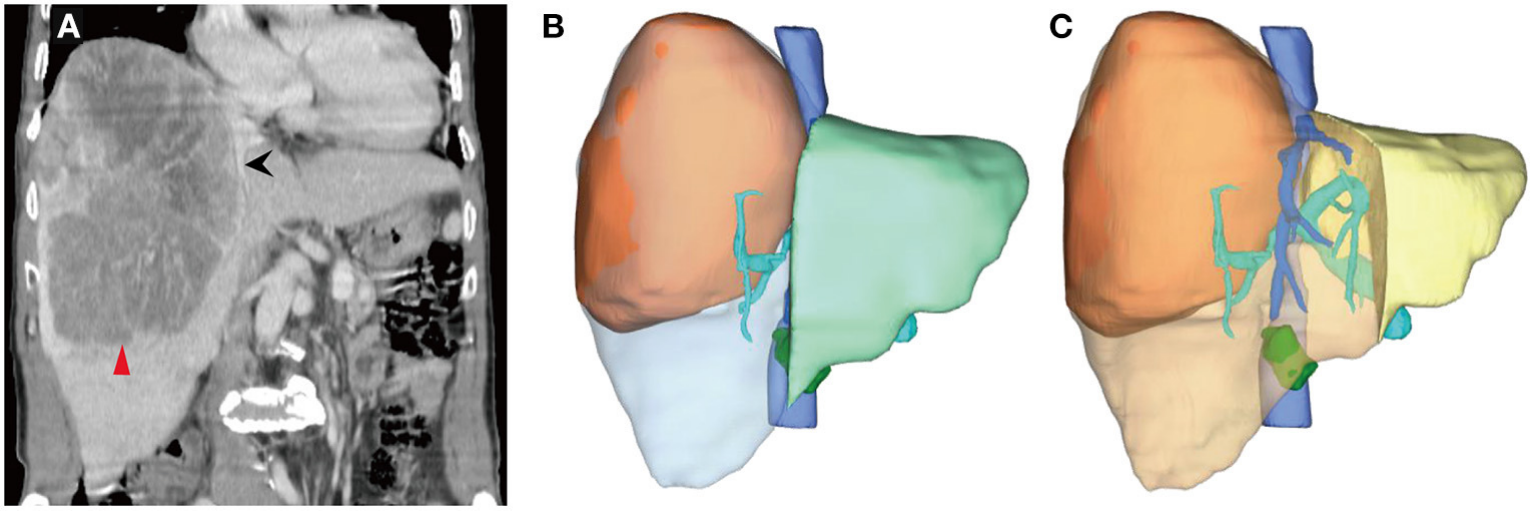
Computed tomography (CT) scans and three-dimensional reconstruction at initial diagnosis. (A) A large lesion (the red arrow head) in the right lobe of the liver, measuring about 19.3 × 14.6 × 13.2 cm. The middle hepatic vein (the black arrow) ran almost along the edge of the lesion. (B) The future liver remnant (FLR, the light green area), followed right hemi-hepatectomy divided by standard liver volume (SLV), was 51.47%, which was well-tolerated for hepatectomy, although the surgical margin was nearly zero. (C) If extended right hemi-hepatectomy were to be performed, the FLR (the yellow area)/SLV would be 25.78%, with an extremely high risk of post-hepatectomy liver failure.
